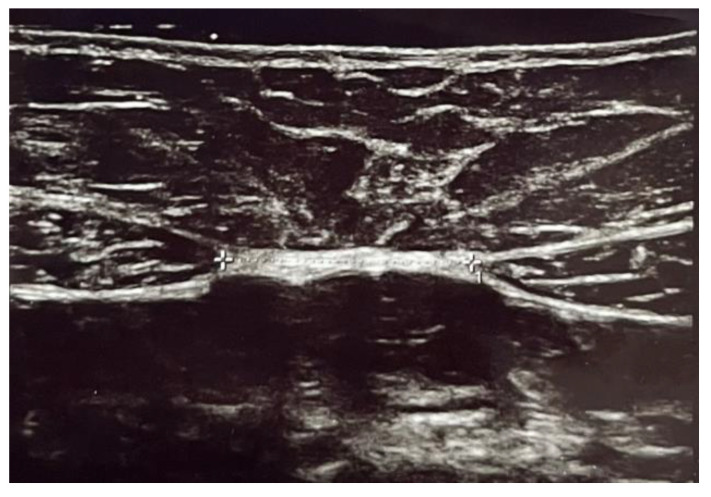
Ultrasound image of subumbilical inter-rectal space.
